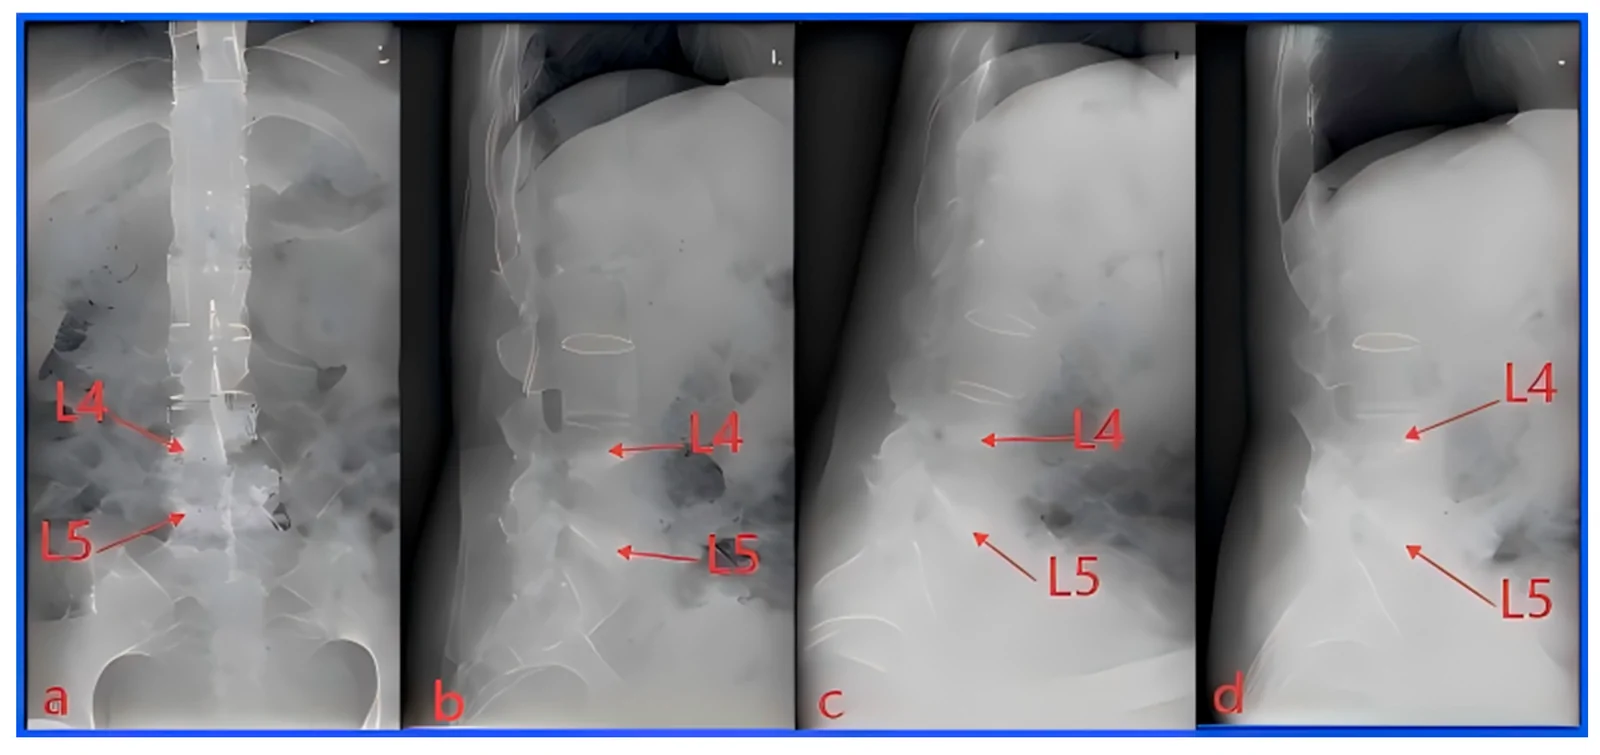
Preoperative lumbar radiographs. (a) Standing anteroposterior radiograph demonstrates the ankylosed “bamboo spine” and the abnormal transverse lesion at the L4/5 level. (b) Standing lateral radiograph shows the L4/5 lesion with anterior column involvement. (c) Flexion radiograph demonstrates no appreciable motion at the L4/5 segment. (d) Extension radiograph likewise shows no appreciable motion at the affected segment despite the structural disruption subsequently demonstrated on computed tomography. Red arrows indicate the L4 and L5 vertebral levels.
